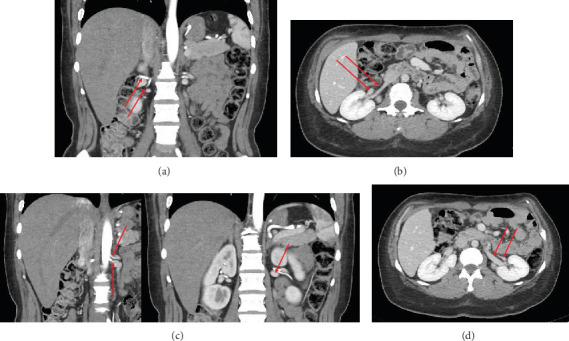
(a) CTA—arteries of right kidney. (b) CTA—veins of right kidney. (c) CTA—arteries of left kidney. (d) CTA—veins of left kidney.
